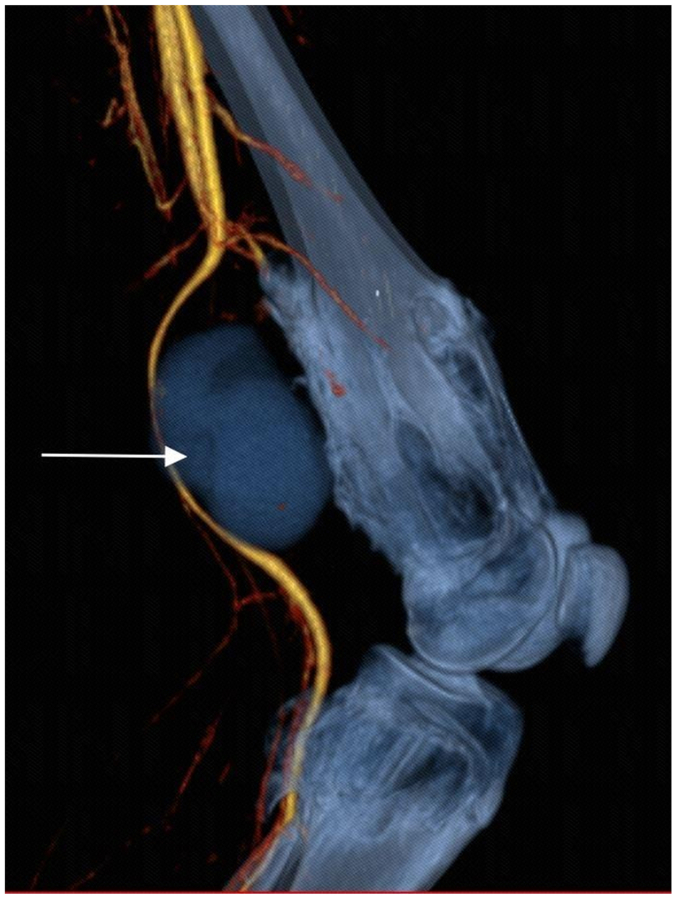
Three-dimensional volume-rendered computed tomography angiography reconstruction of the left knee. The *white arrow* indicates the popliteal artery pseudoaneurysm (*blue mass*) adjacent to the distal femoral metaphysis.
